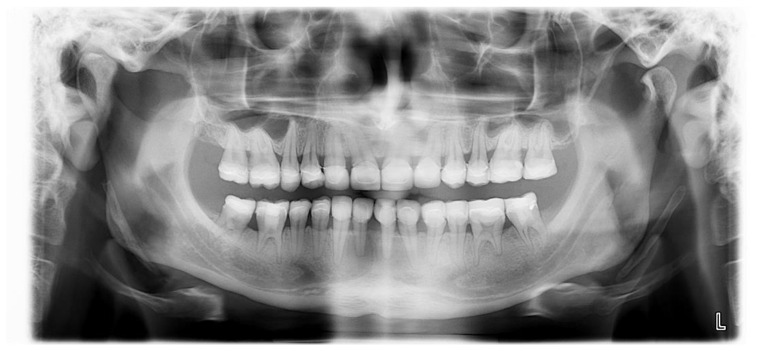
Orthopantomogram of the patient at 8 months after the end of the dental treatment.
